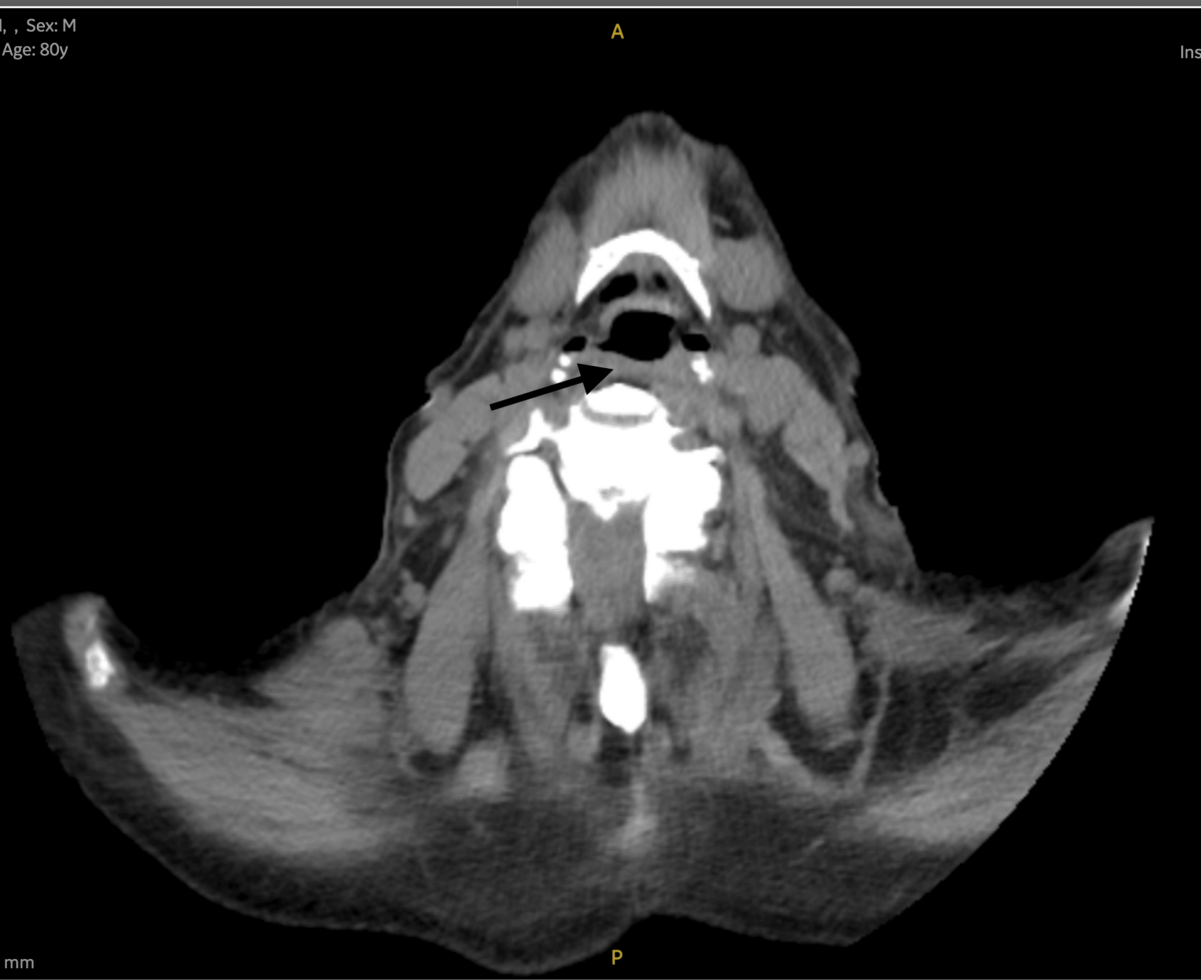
Post-rupture CT scan of the neck, axial view, confirming absence of the cyst (black arrow) and normalization of the hypopharyngeal contour
